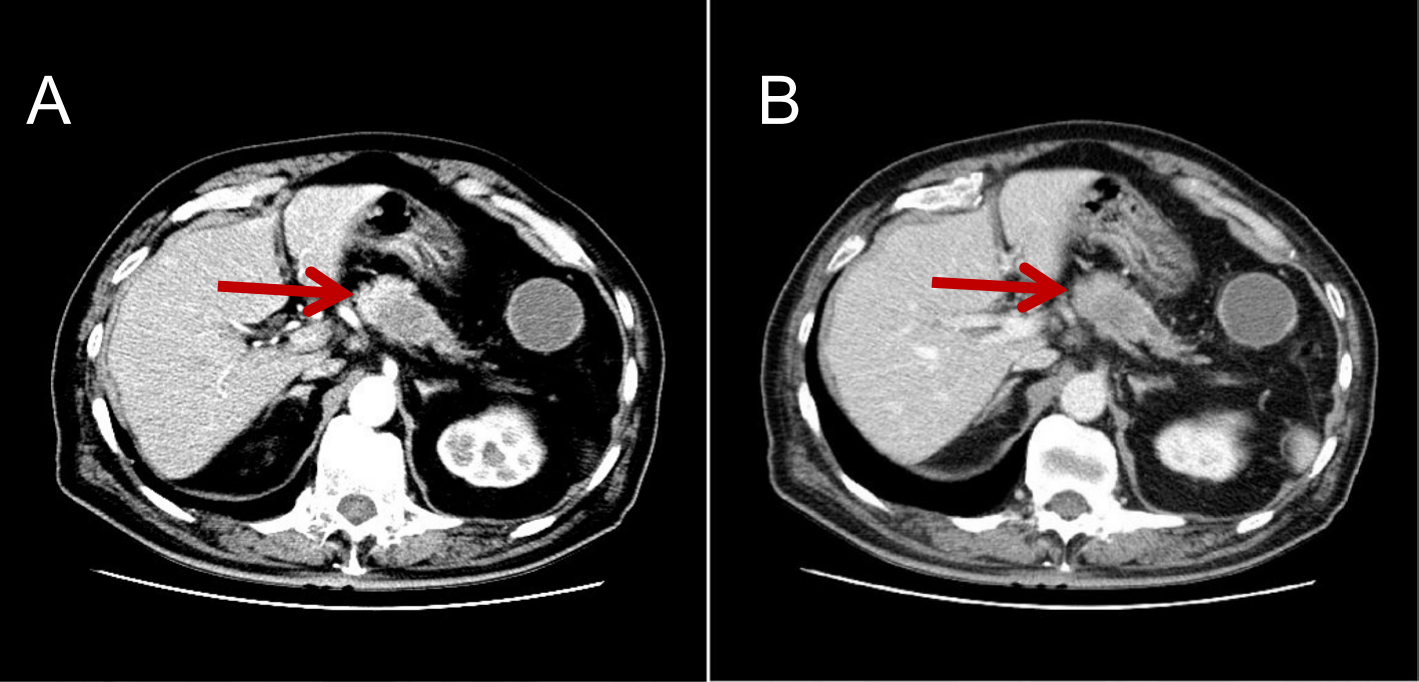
Abdominal CT scan showing a 5.5 × 2.8 × 2 cm mass in the pancreas (indicated by the arrow). A Marginal enhancement of the arterial area. B No enhancement in the venous area
